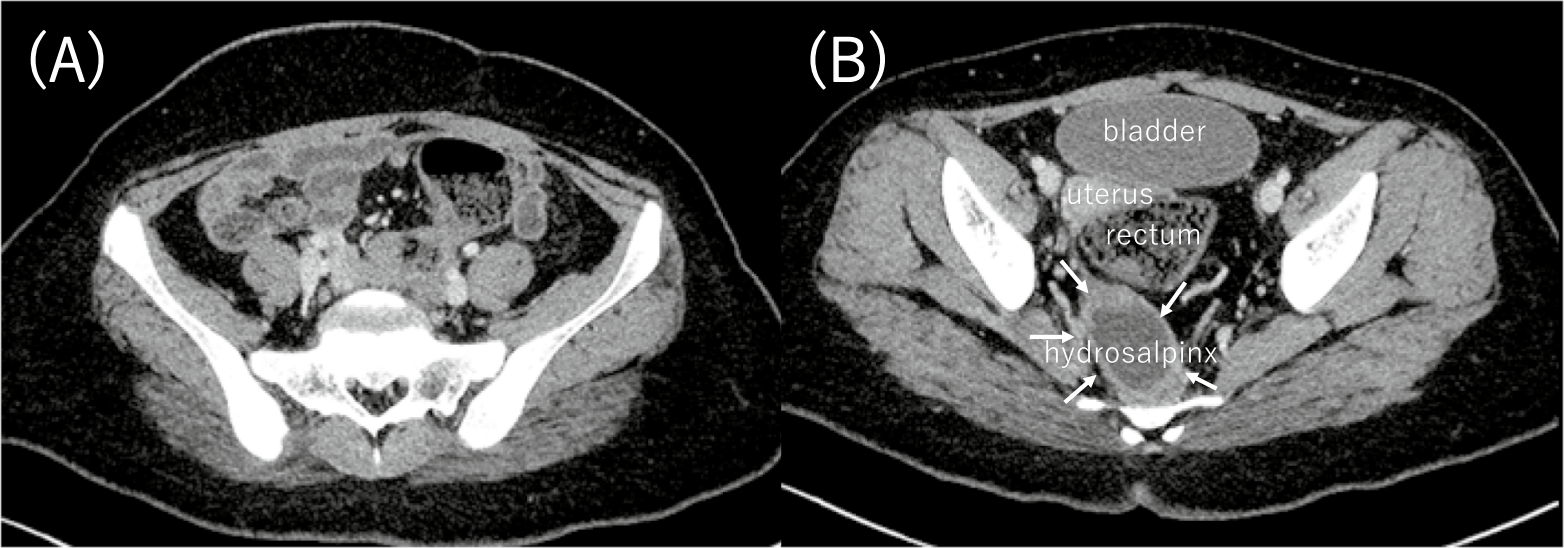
CT. A Abdominal abscess and pyosalpinx disappeared. B Hydrosalpinx was remained in the pelvis
